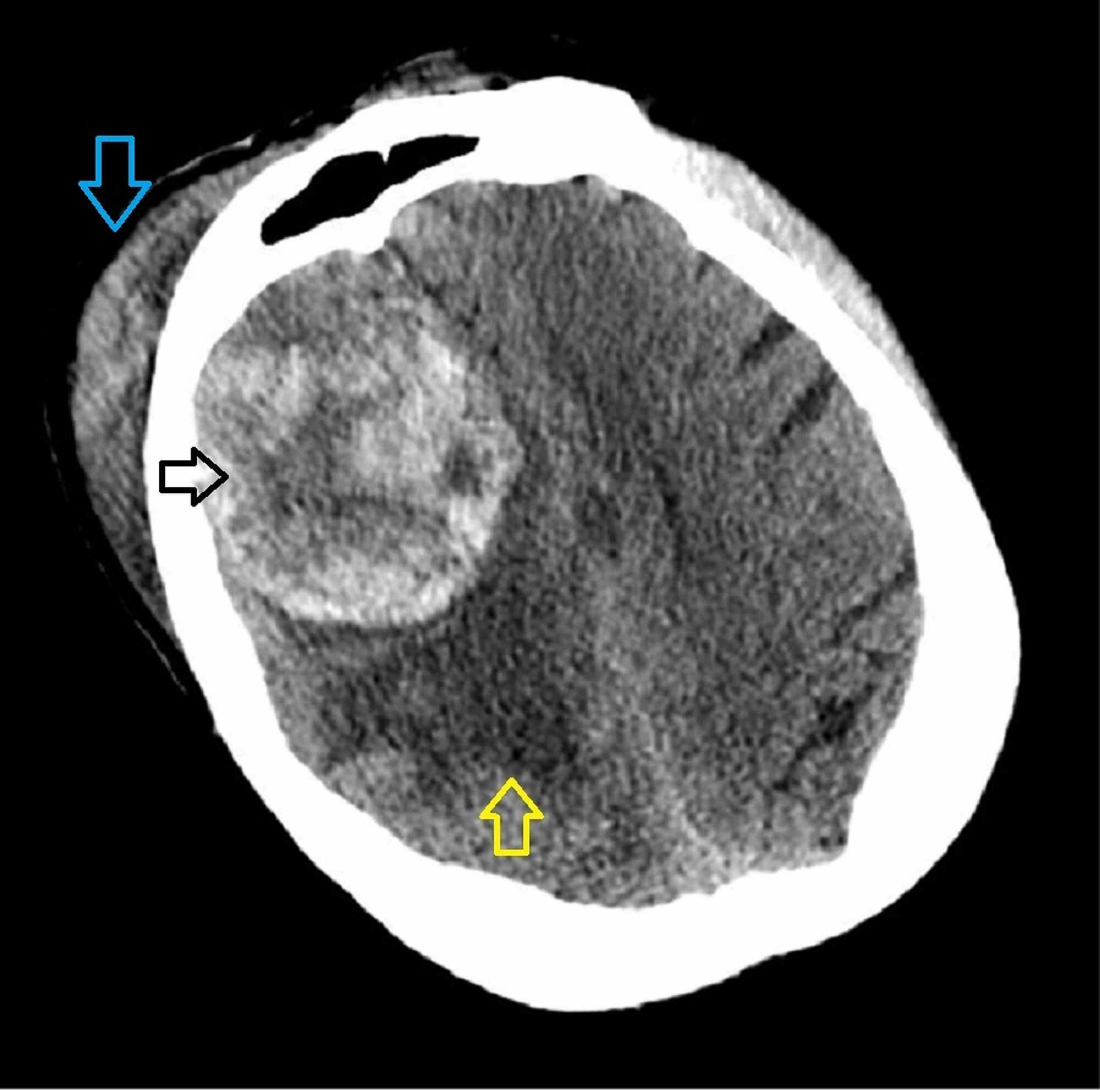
Pre-operative non-contrast CT head Axial cut at the level of the frontal sinus demonstrates a large right frontal, extra-axial, heterogeneously dense mass measuring 5.7cm x 6.5cm x 5.0cm in size (black arrow). The mass is surrounded by notable vasogenic edema (yellow arrow) causing midline shift and subfalcine herniation. A right frontal subgaleal hematoma is evident without adjacent skull fracture (blue arrow).
